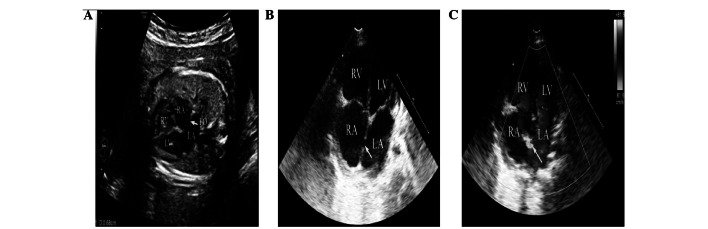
Comparison of antepartum foramen ovale (FO) size and puerperal atrial septal defect. (A) The diameter of the FO was 6.8 mm at 24 weeks; (B) two dimensional echocardiography revealed an atrial septal defect at 12 months after birth; (C) color Doppler flow imaging revealed a left to right atrial shunt. RV, right ventricle; RA, right atrium; LV, left ventricle; LA, left atrium.
